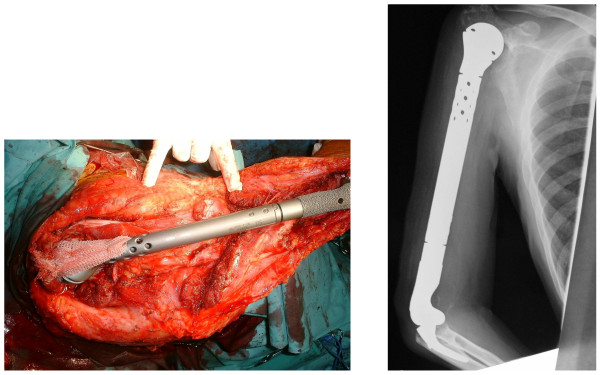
Intraoperative findings and a postoperative plain radiographics images. Total humerus replacement was performed, and an HMRS artificial joint for tumor treatment was inserted and hung using an artificial ligament. We used the Leeds-Keio as the artificial ligament for this procedures. The ISOLS radiographics evaluation was excellent for all items.
